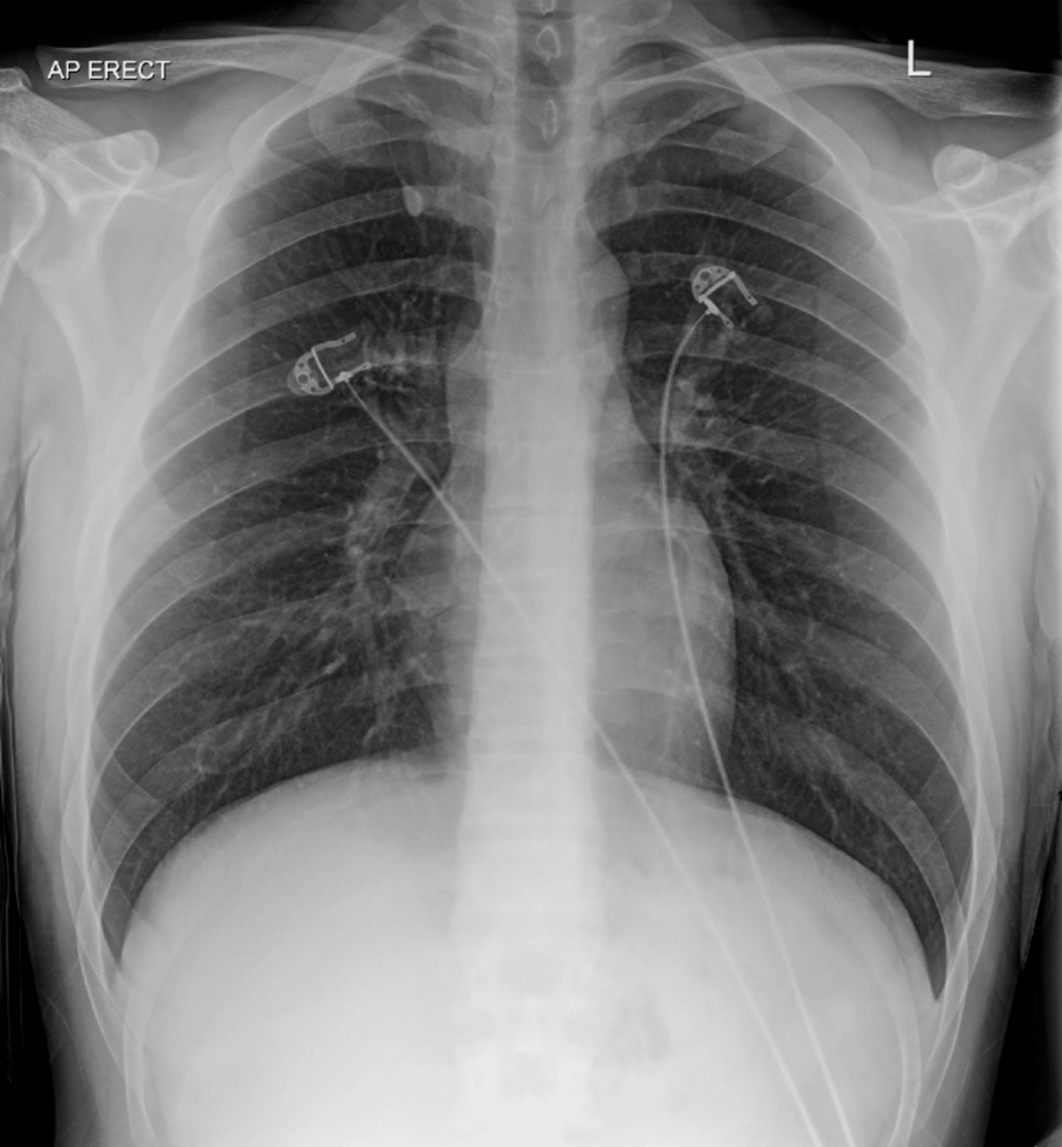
Follow-up chest X-ray showing the resolution of surgical emphysema or pneumomediastinum.
